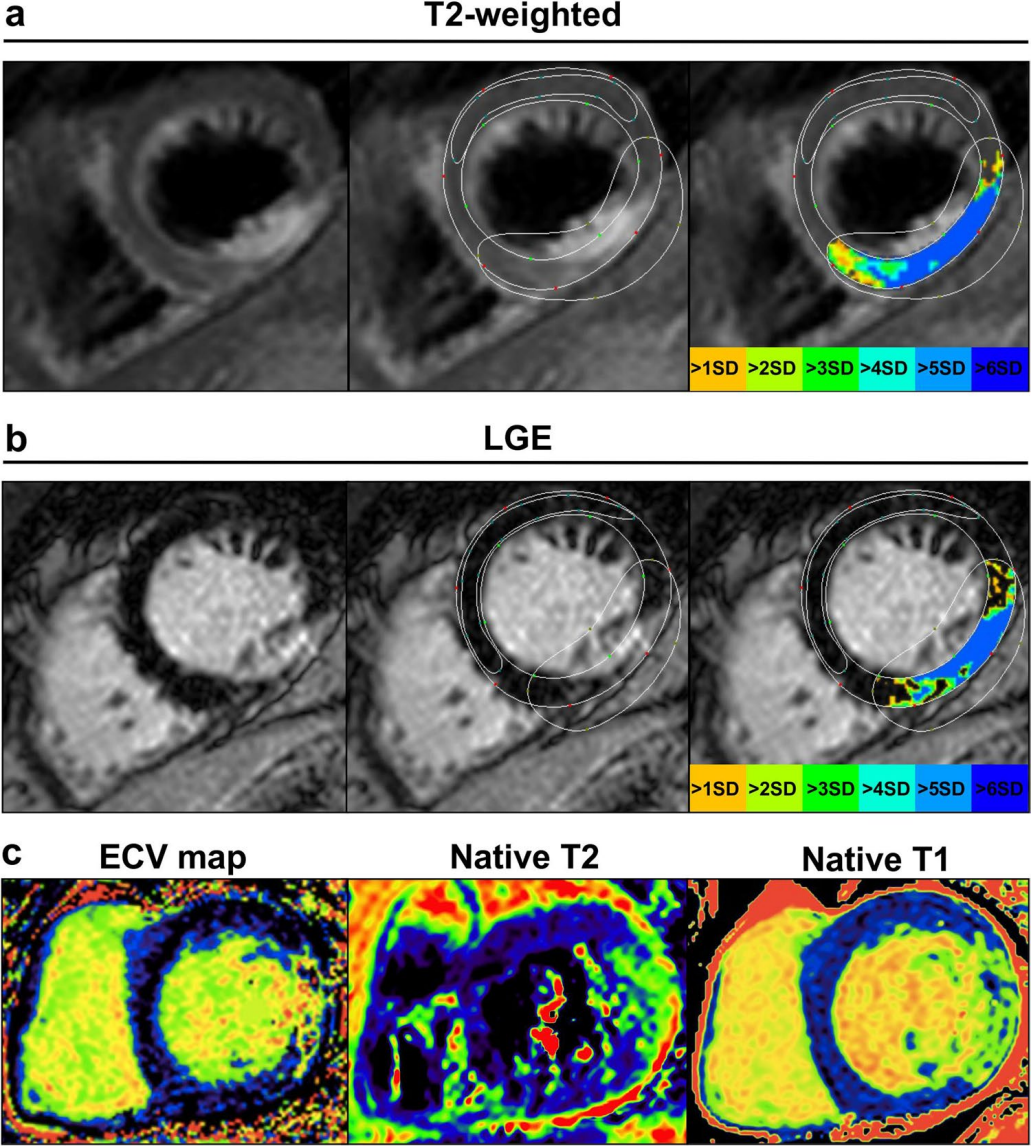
Cardiac T2-weighted CMR image (a), LGE image (b), ECV, native T2 and T1 maps (c). For quantitative analysis of lesion size, a large region of interest (ROI) was placed in remote myocardium to quantify mean signal intensity or mean relaxation times, including standard deviations as shown in a and b. The threshold was set at >2 SDs to define lesion size in the infarct area. Care was taken not to include the blood volume or the epicardial fat in the measurements by precise placement of the endo- and epicardial myocardial borders, as shown in the colored T2-weighted and LGE images. ECV, extracellular volume; LGE, late gadolinium enhancement; SD, standard deviation
